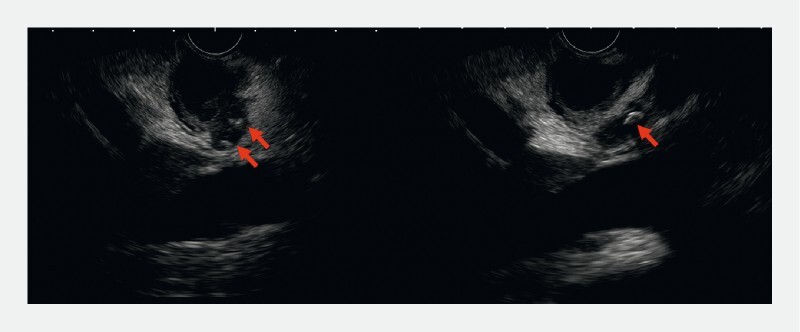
Endoscopic ultrasonography images showing a distended gallbladder, cholecystolithiasis, and suspected choledocholithiasis.
